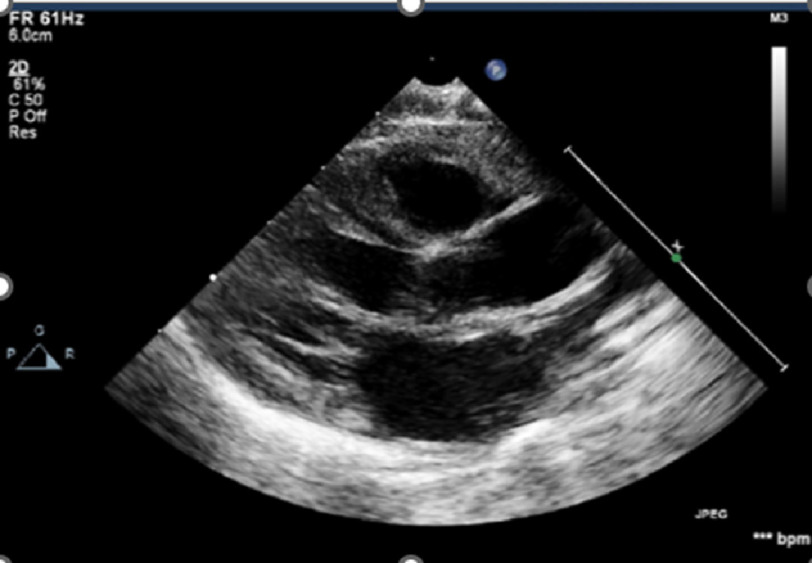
Transthoracic echo, parsternal long axis view shows recovered LV dimensions and function postablation.
